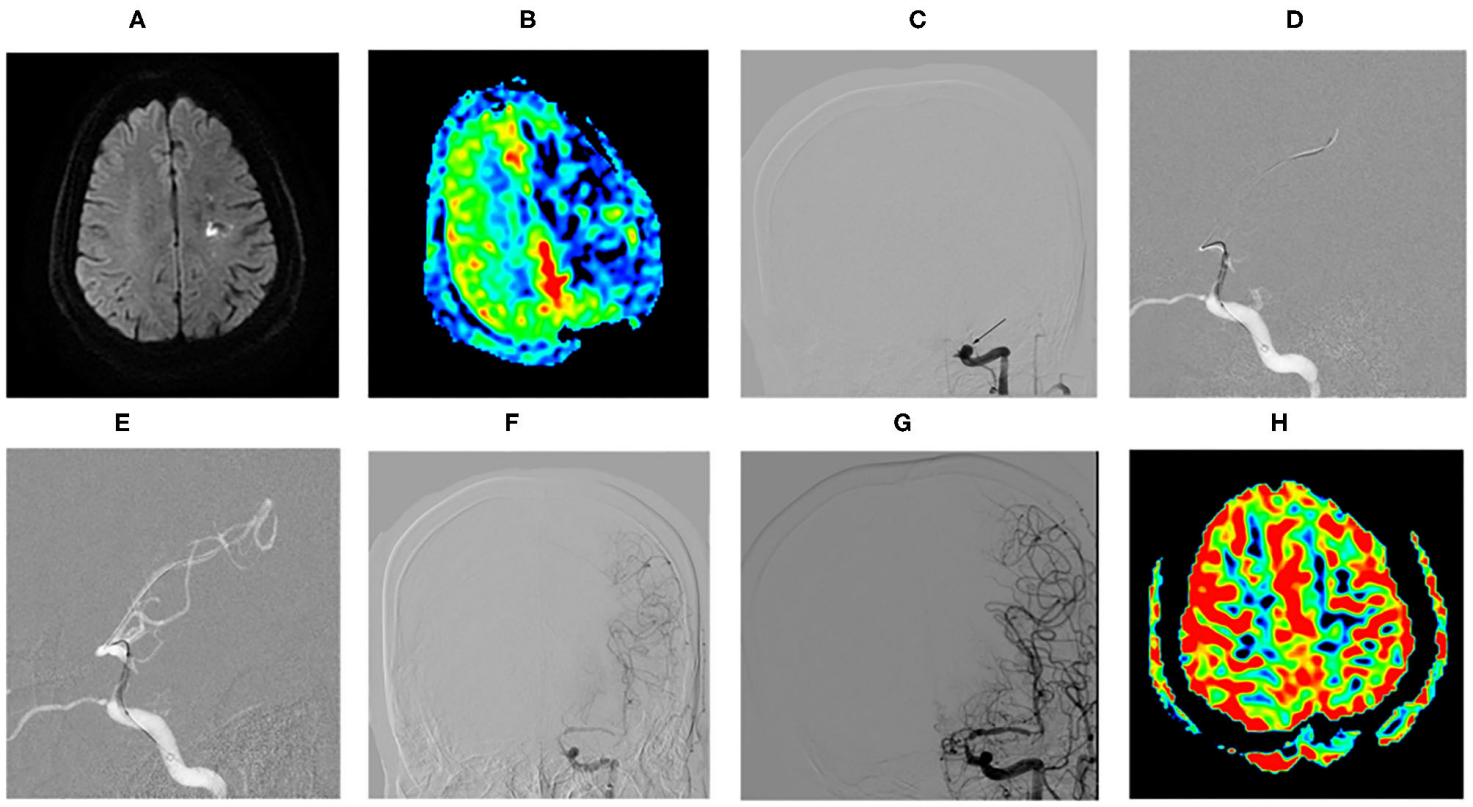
Example of comparing the preoperative MRI and operative angiographic findings with follow-up. (A) MRI revealed left watershed infarction. (B) ASL showed left cerebral hemispheric hypoperfusion in the ICAO territory. (C) DSA confirmed the left internal carotid artery terminus occlusion ipilaterally to the infarcts (arrow). (D) Predilatation with a conventional balloon. (E) DCB dilatation after predilatation. (F) DSA showed the occlusion recanalization with 70% residual stenosis with a large number of thrombus (arrow). (G) DSA showed thrombus disappeared and residual stenosis rate improved with 50% residual stenosis at angiographic follow-up of 1 month (arrow). (H) ASL showed basically symmetric bilateral cerebral hemispheric perfusion at angiographic follow-up of 1 month.
